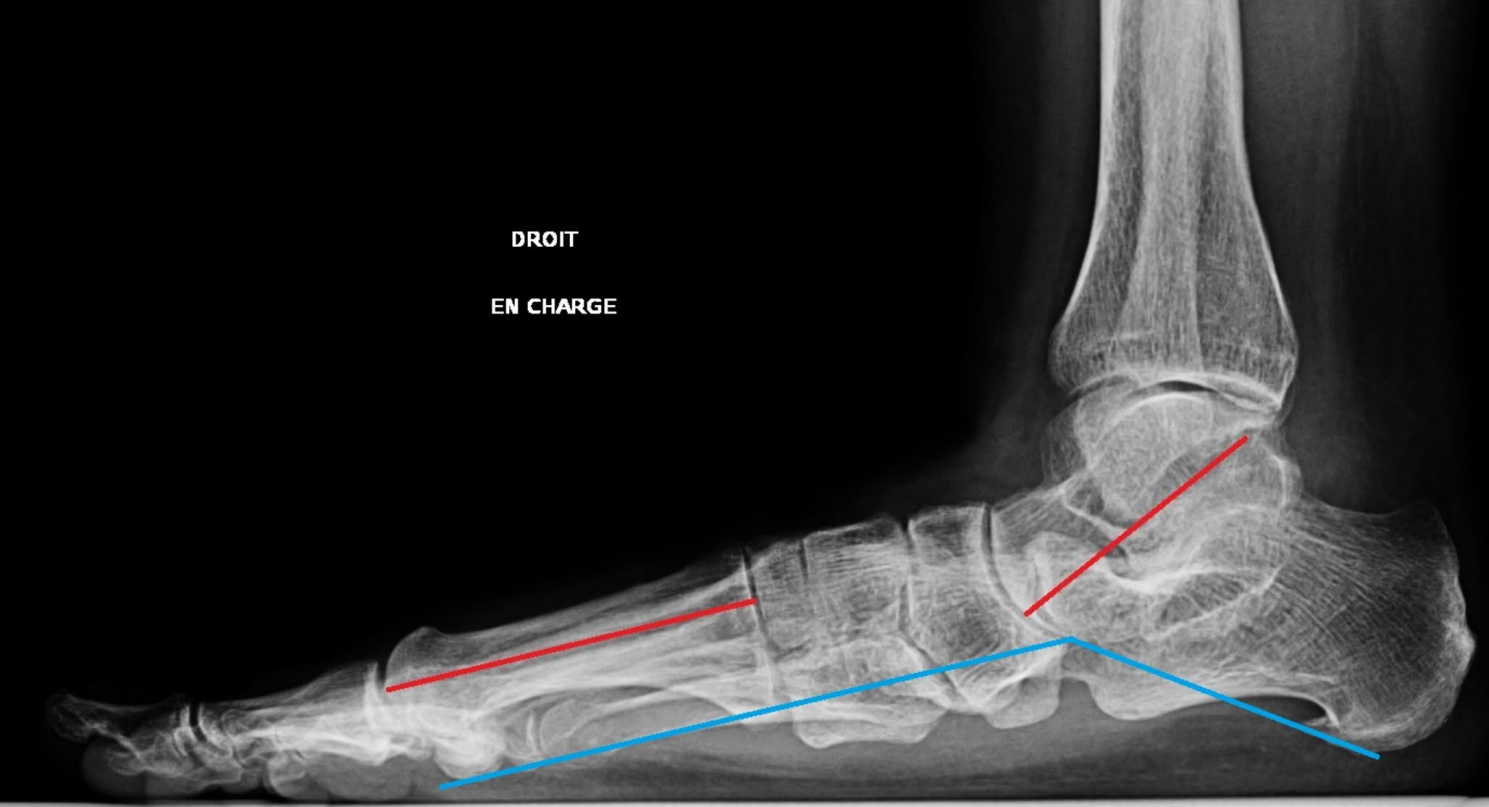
Lateral weight-bearing radiograph of the right foot. Talus–first metatarsal axis in red and plantar arch angle in blue
